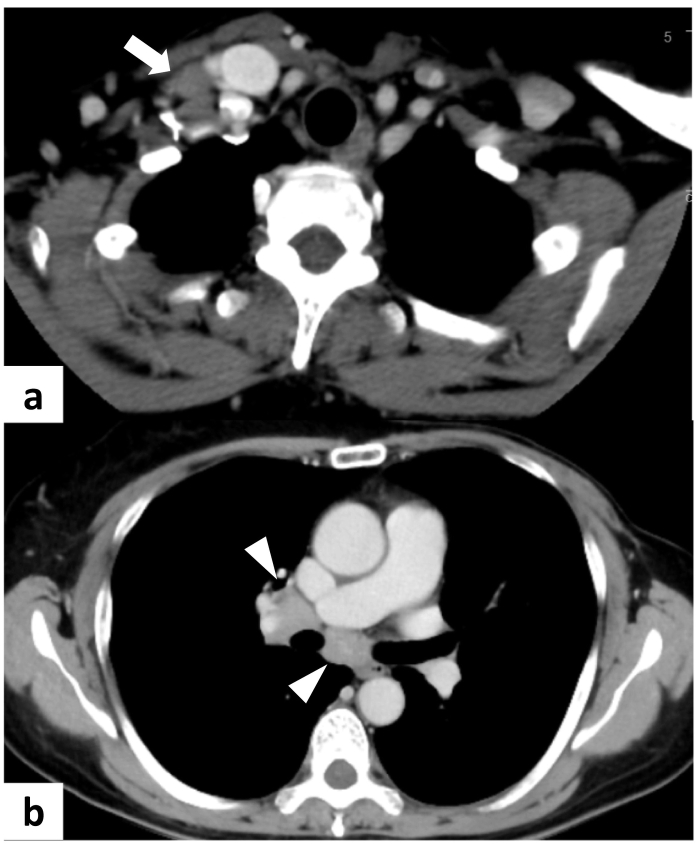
a: A contrast-enhanced chest CT scan showing enlarged lymph nodes located in the right supraclavicular region (arrow). b: The enlarged lymph nodes were also visualized in the right hilar and subcarinal regions (arrow heads).
